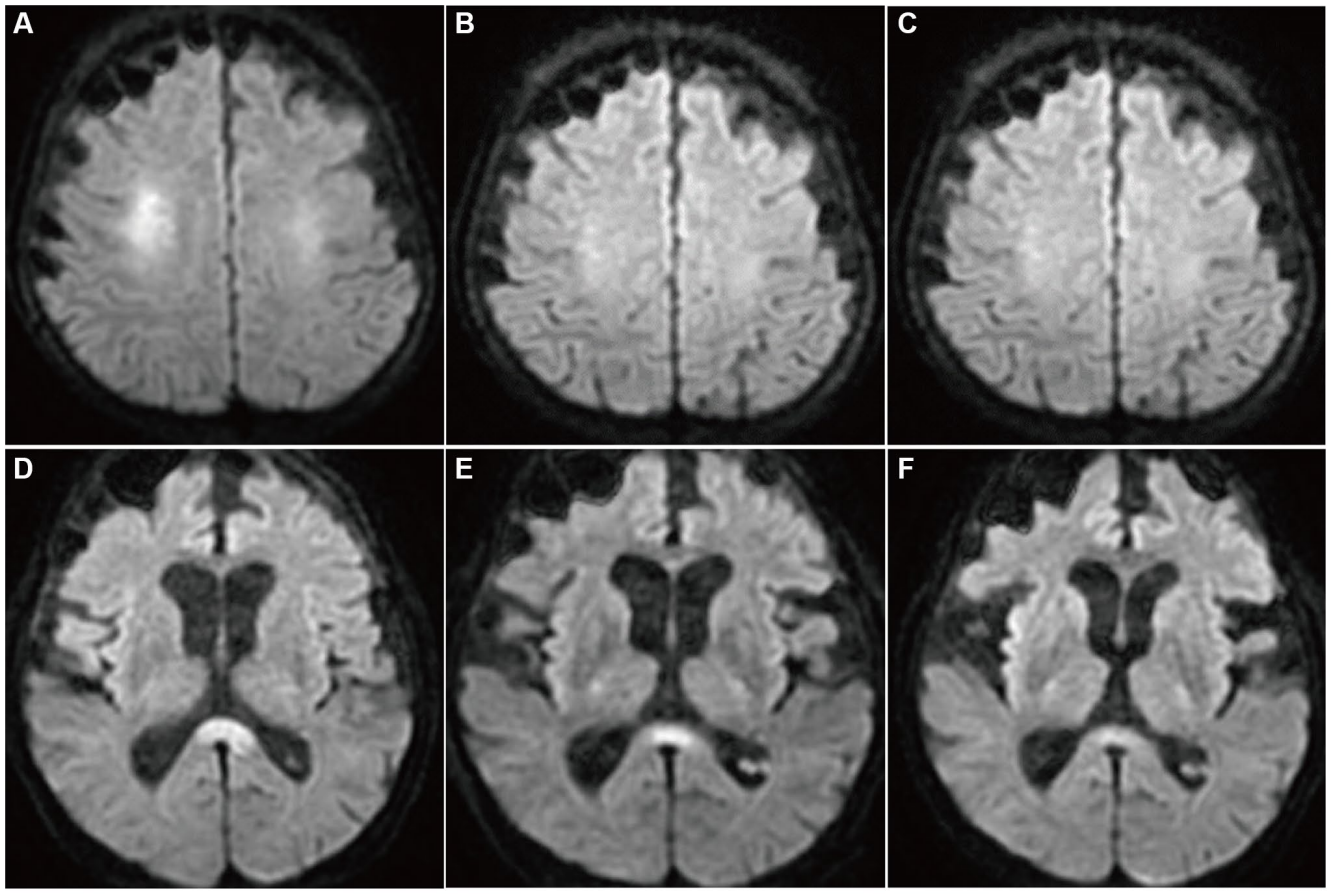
Diffusion-weighted brain MRIs (DWI). On day 2 of admission, hyperintensity was noted in the bilateral deep white matter and the corpus callosum splenium (A,D). On days 3 (B,E) and 6 (C,F), the hyperintensities were attenuated gradually.
